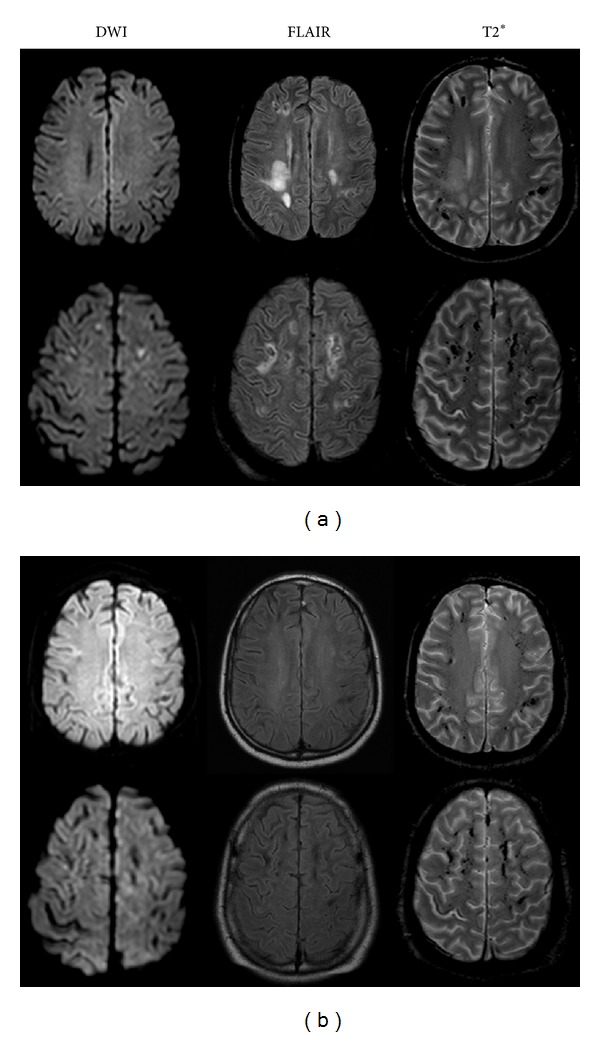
MRI manifestations of Patient 3. (a) Deep white matter edema in frontoparietal lobe with hemorrhagic lesions. (b) Resolution of brain edema with residual hemorrhagic lesions.
